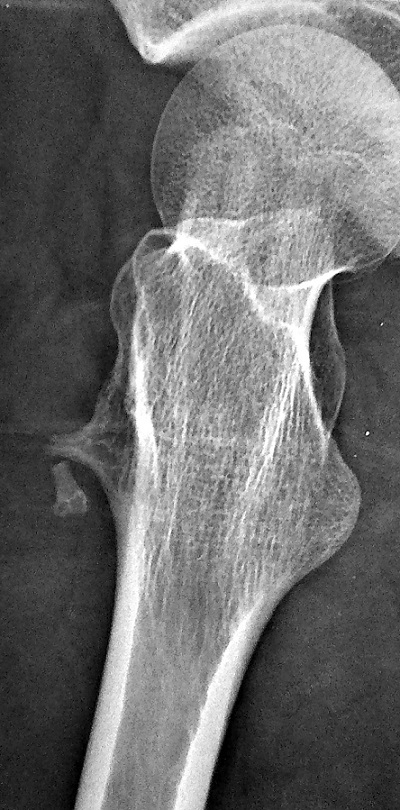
Radiographie du fémur droit montrant l'exostose pédiculée à l'extrémité supérieure de la diaphyse fémorale compliquée d'une fracture
